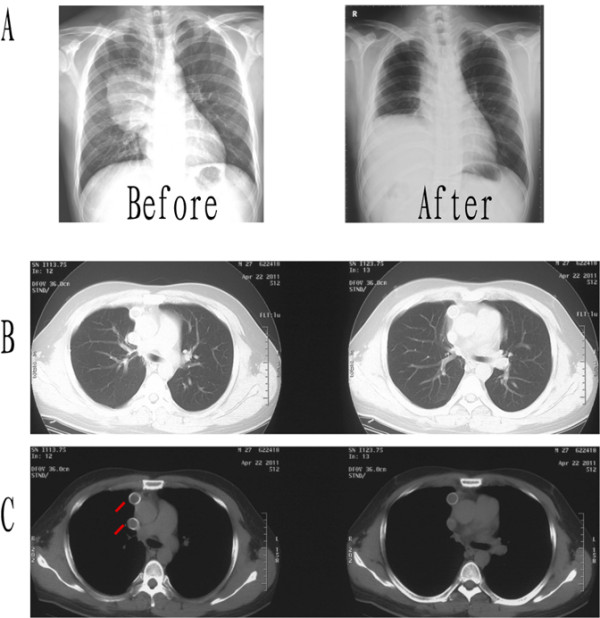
Chest X-ray and CT scan after operation. (A) The chest x-ray images of the patient before/after operation. (B and C) The chest CT scan 16 months after the operation. The red lines show the SVC replacement graft.
